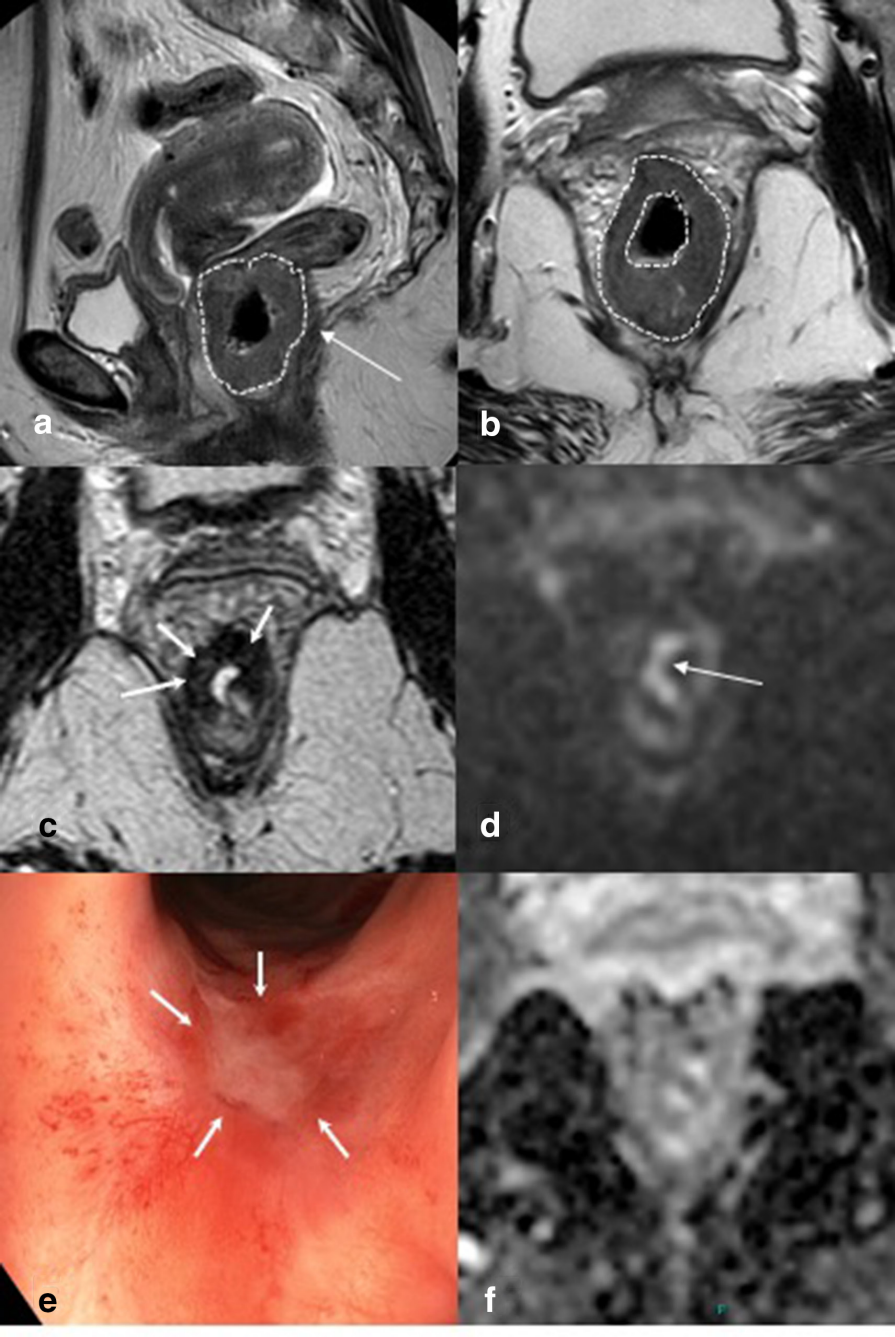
Near complete response. (a/b) Distal tumour outlined with dotted line with extramural extension on sagittal and axial T2-weighted imaging with invasion into the levator muscle (arrow in a). (c) After chemoradiation on axial T2-weighted imaging, a full thickness fibrosis is found with heterogeneous signal (white arrows in c). Endoscopy (e) shows an almost completely healed scar with central depression and a flat ulcer <1 cm (white arrows), surrounded by teleangiectasia. On DWI and ADC map (d/f) no restriction is found, only luminal T2 shine through (white arrow). ADC, apparent diffusion coefficient; DWI, diffusion-weighted imaging.
